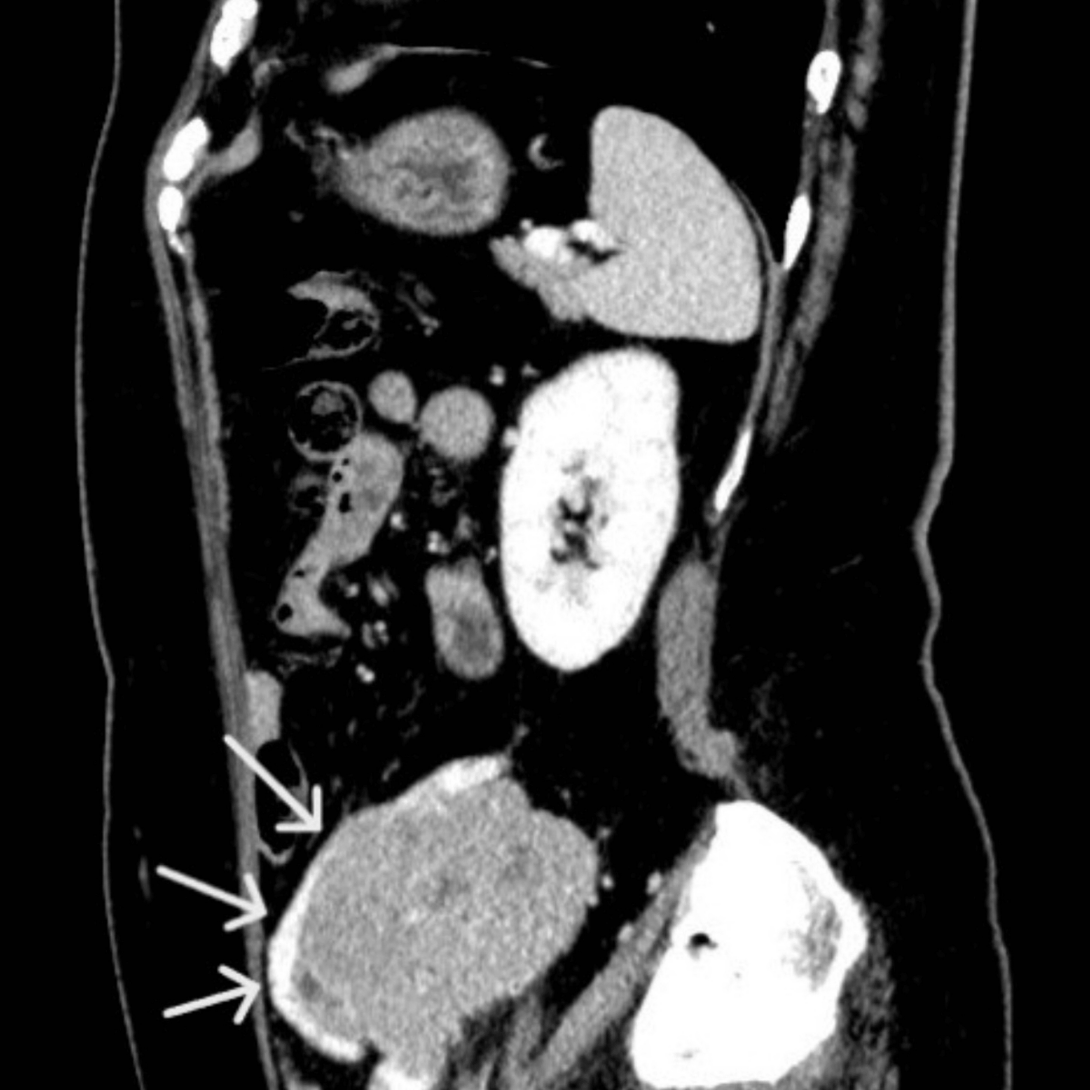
Contrast-enhanced CT (sagittal view). CT showing the left ovarian vein (white arrow) draining into the mass, suggesting an ovarian origin, with areas of poor enhancement indicating necrosis.
